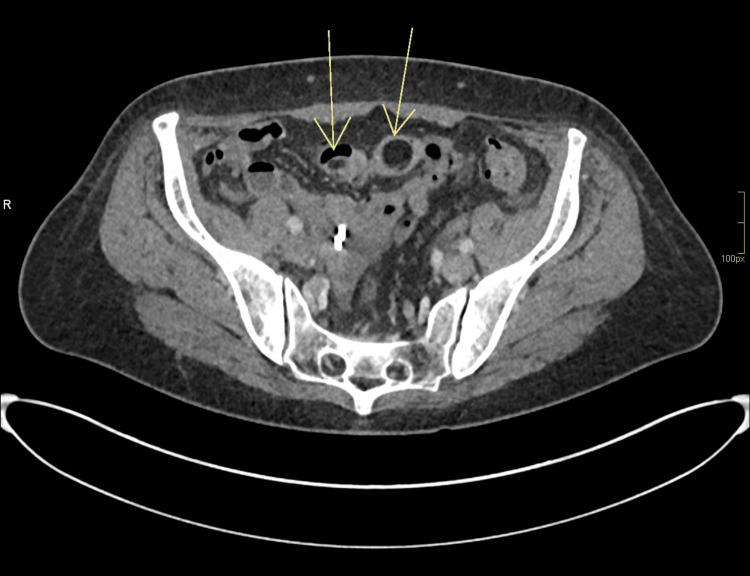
Axial view of the polyp shown on CT scan abdomen with contrast
